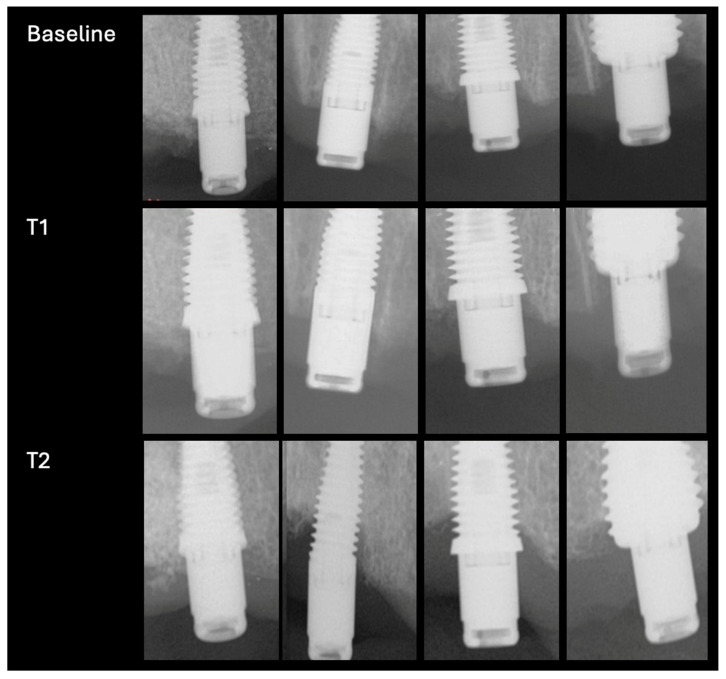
Radiographs of a typical case at baseline, at follow point T1 and at follow up point T2.
